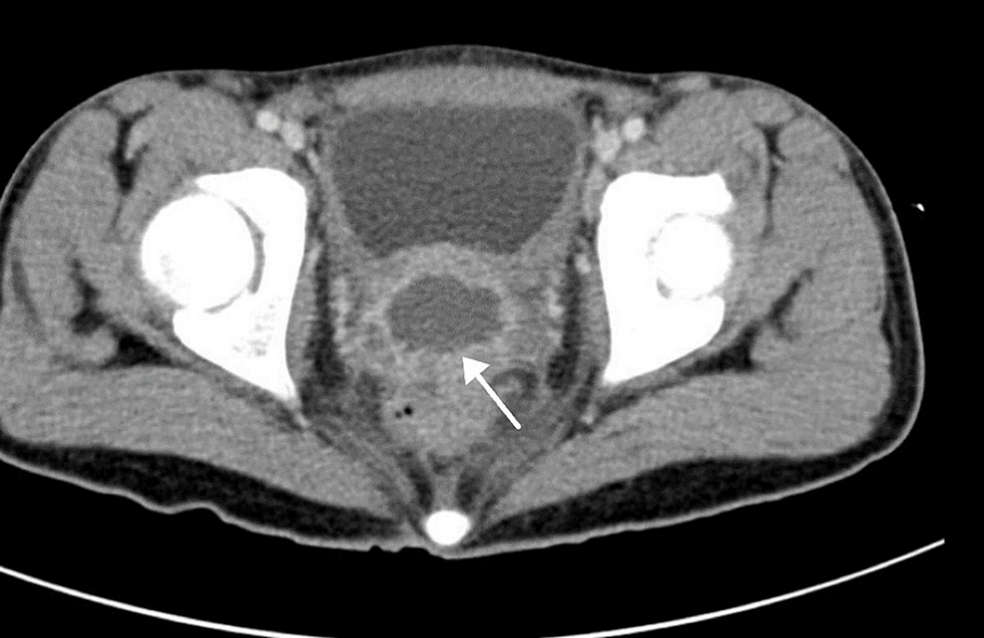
Computed tomography (axial view) revealing multilocular and septated prostatic cyclic lesion measuring approximately 5 × 4 × 3 cm that could be a cystadenoma or prostatic abscess
